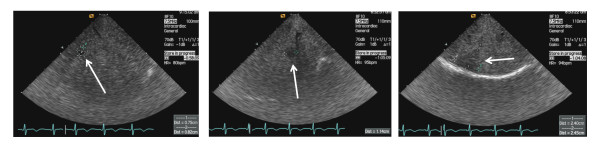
Endovascular ultrasound view of focal lesions in a cirrhotic liver. With high resolution the visualization of small sized nodules (diameter 0.82 cm, left panel, and 1.14 cm, central panel) was possible. The right panel shows a larger hypoechogenic lesion located in the sub-capsular region of a multinodular liver. Arrows indicate in each case the described lesions.
